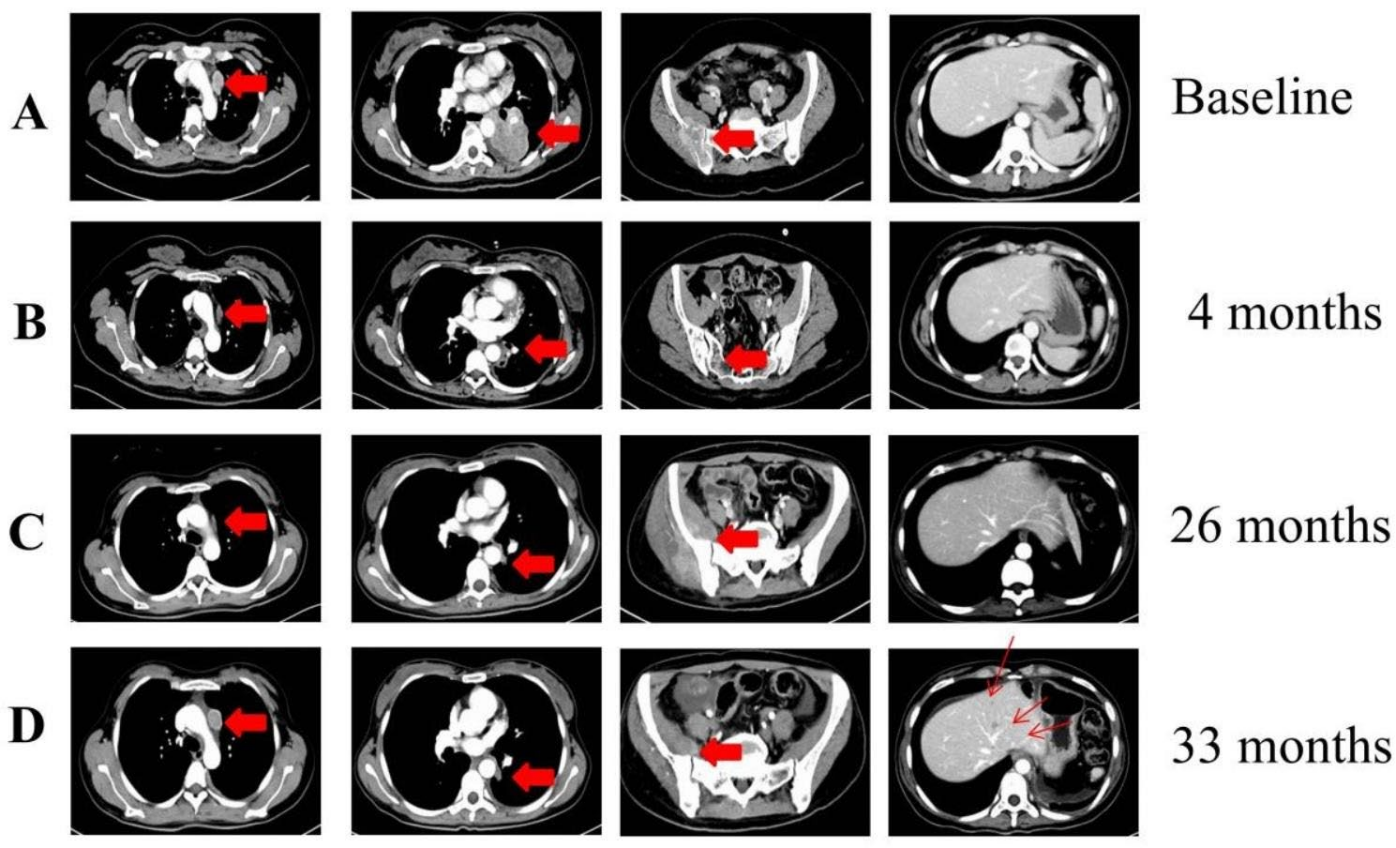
Imaging in diagnosis and treatment. An enhanced CT image of this patient at follow-up. (A) At initial diagnosis. Arrows indicated mediastinal lymph nodes, left lung masses, and soft tissue metastases of the right ilium, but no lesions in the liver. (B) After 4 months, the mediastinal lymph nodes, right lung, and right iliac crest lesions became significantly smaller and was assessed as PR. (C) After 26 months, the right iliac lesion was enlarged, but the rest of the lesions were not. Assessed as oligoprogression. (D) 33 months after taking selpercatinib, the mediastinal lymph nodes were enlarged and multiple intrahepatic metastases appeared
